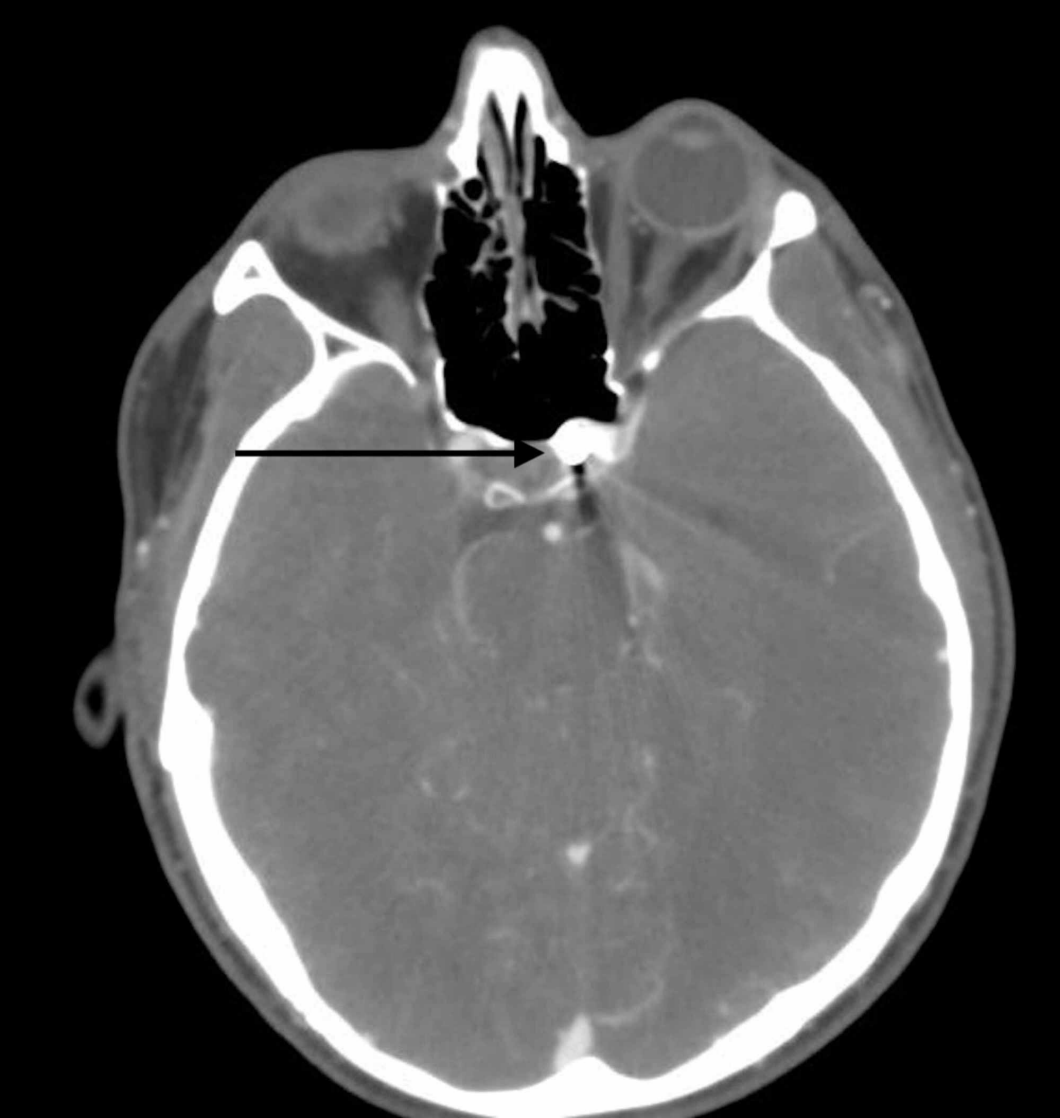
CTA of the injury The image shows the nail traversing the left internal carotid artery in the cavernous sinus in the carotid siphon region (black arrow) CTA: computed tomography angiogram
